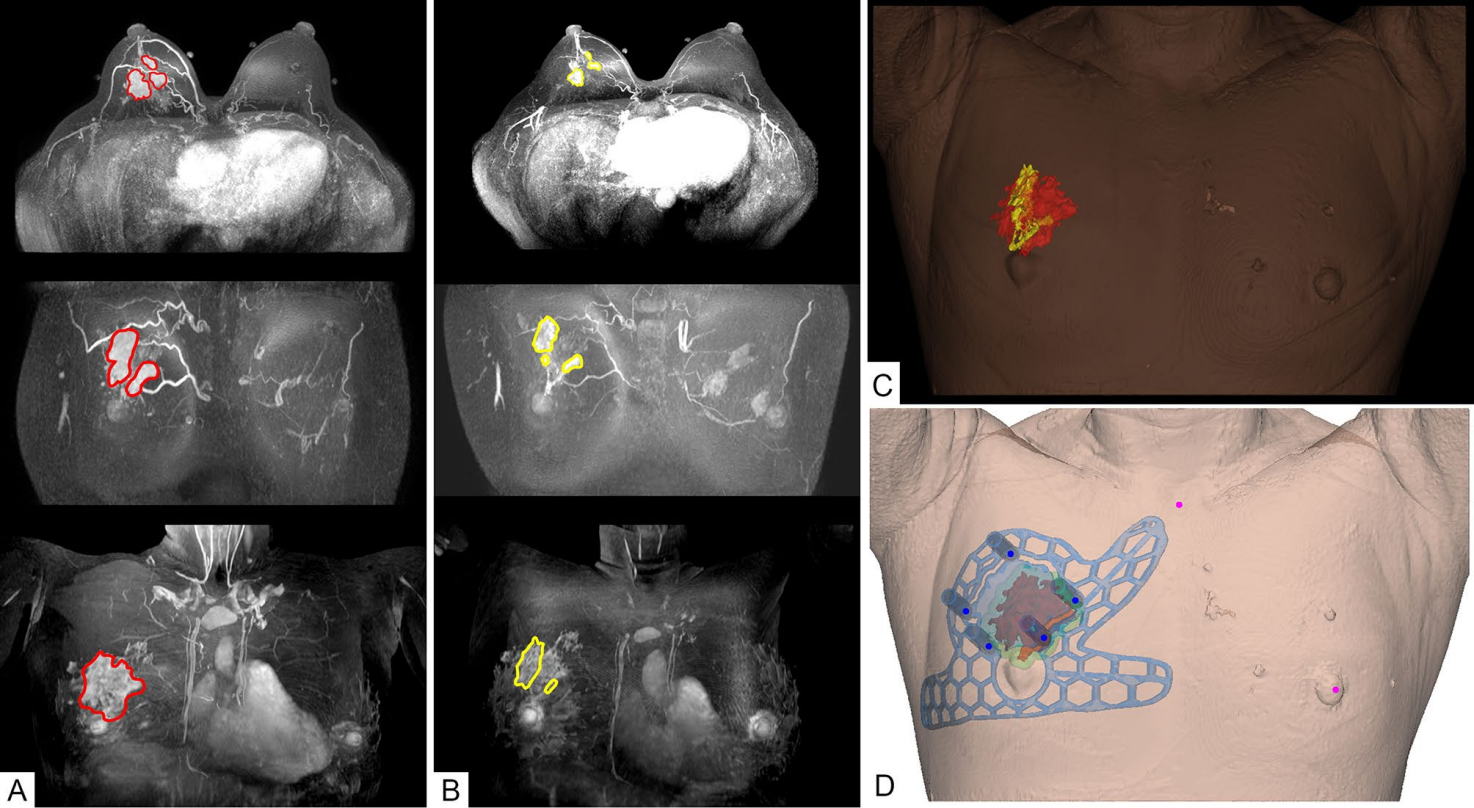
Three-dimensional modeling of the breast and tumor area based on magnetic resonance imaging (MRI) in neoadjuvant systemic therapy (NST) patient and patient-specific tumor target 3D printed-breast surgical guide (3DP-BSG) modeling. (A) 3D modeling of the breast and tumor area using MRI before NST. (B) 3D modeling of the breast and tumor area using MRI after NST. (C) 3D modeling of the breast and tumor area using MRI fusion of the images obtained before and after NST. D. Patient-specific 3D-BSG modeling using three-dimensional information about breasts and tumors.
